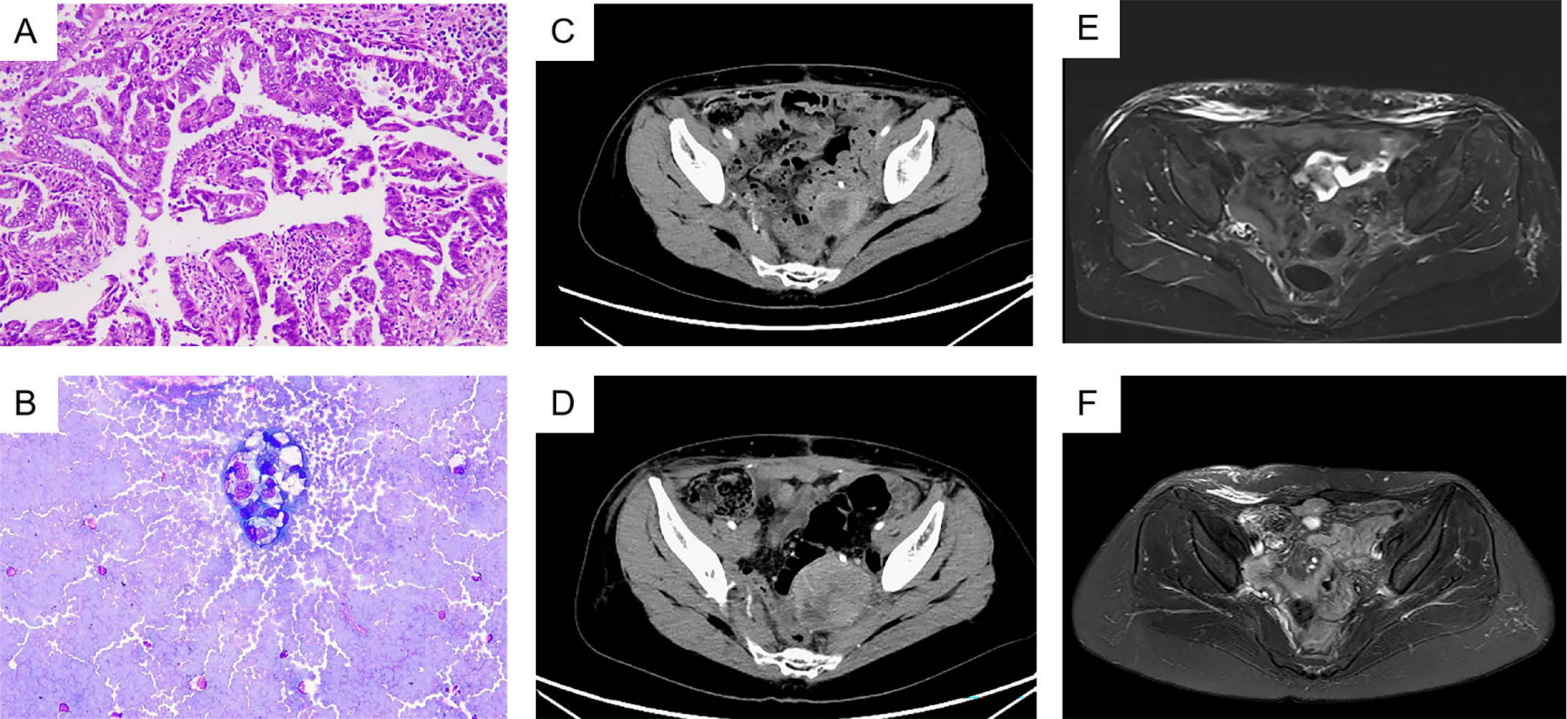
(A) HemateinEosin (B) Heterotropic cells were seen in ascites. (C) 2022-9-27 Total abdominal computerized tomography (CT). (D) 2022-10-25 Total abdominal CT, progressive disease. (E) 2023-1-4 Total abdominal magnetic resonance, complete remission has been achieved. (F) 2024-4-7 Total abdominal MRI, sustained complete response.
